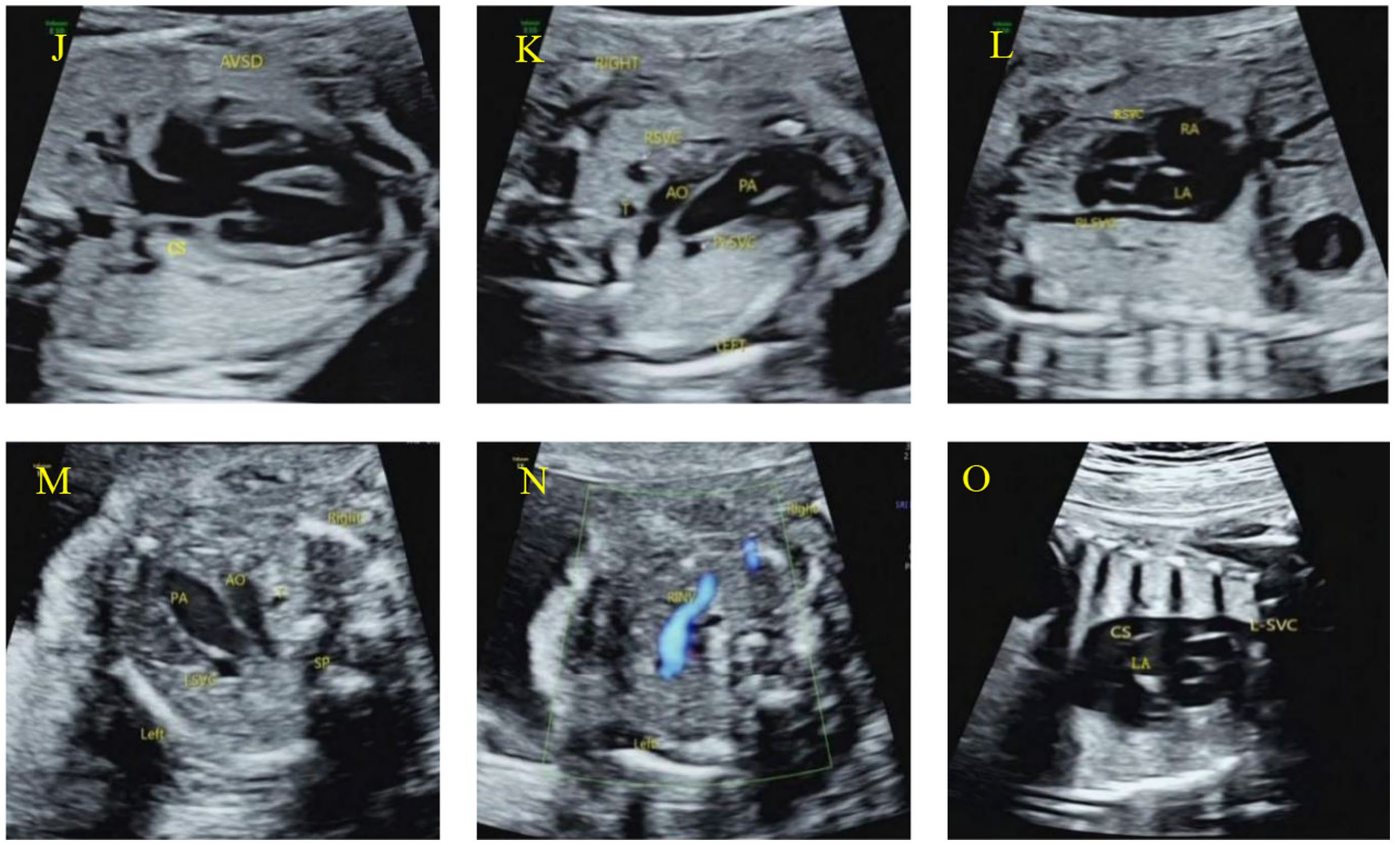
Characteristic ultrasound images of Type II PLSVC: (J–L) and (M-O). Type IIb: (J) The 4CV section shows atrioventricular septal defect and coronary sinus; (K) the 3VT section shows the left superior vena cava on the left side of the pulmonary artery; (L) the paravertebral sagittal section of the neck and chest shows the left superior vena cava draining into the left atrium. Type IIc: (M) The 3VT section shows the left superior vena cava on the left side of the pulmonary artery, and the right superior vena cava is not shown; (N) CDFI shows the right innominate vein draining into the left superior vena cava on the innominate vein section; (O) the paravertebral sagittal section of the neck and chest shows the left superior vena cava draining into the left atrium.
